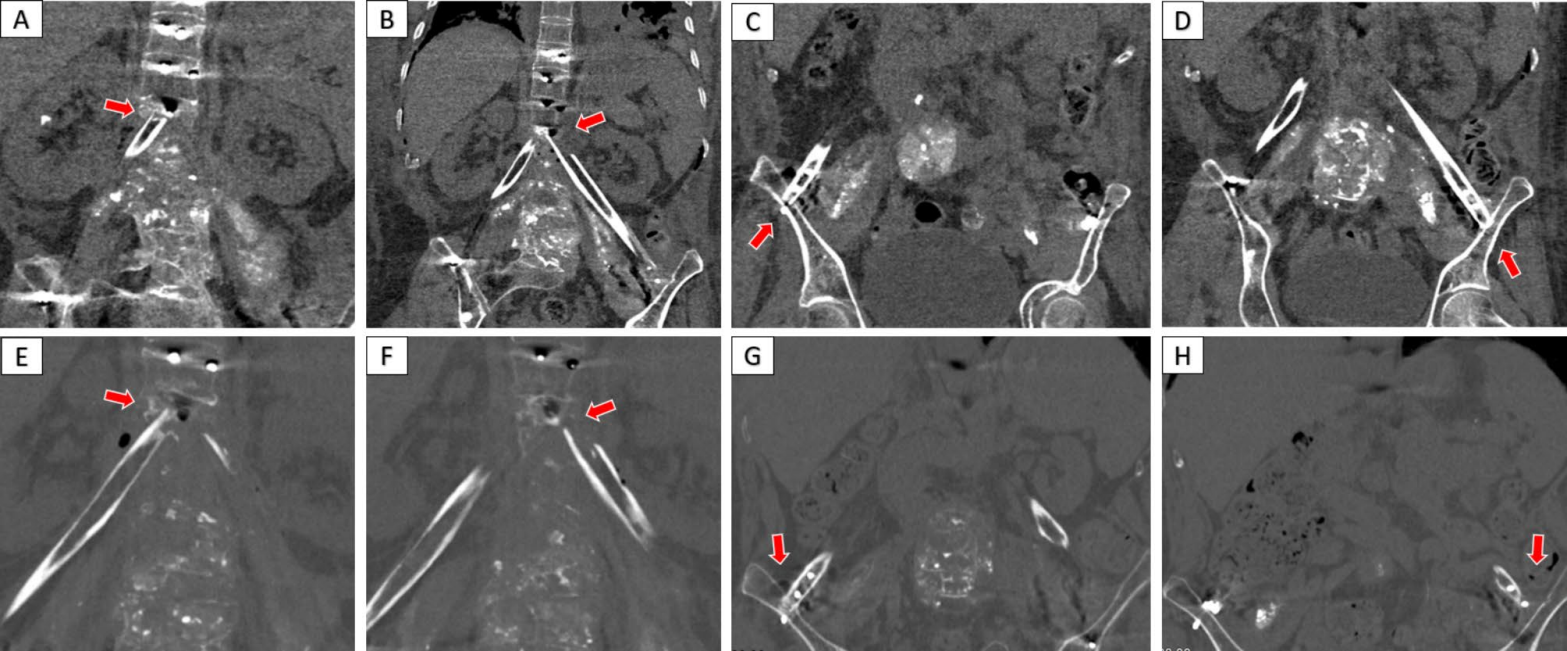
(A-D) After the second stage, CT reexamination showed that the bilateral fibula was fixed with the lower edge of the T12 vertebral body and the upper edge of the iliac spine. (E-H) During the 5-year postoperative follow-up period, there was no significant subsidence of the fibula at the fixation site compared to Figures A-D.
